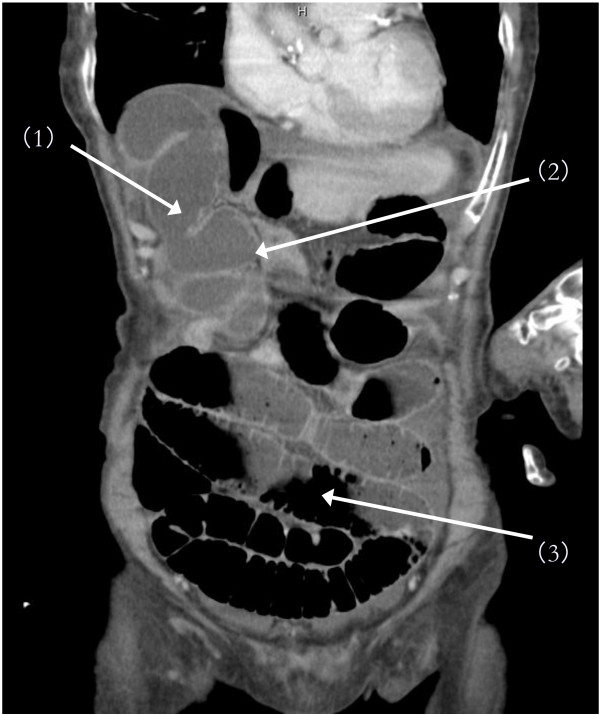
Pre-operative abdominal helical computed tomography (CT): coronal view. A membranous structure (1) was visualized in the center of the upper abdomen between the diaphragm and the left lobe of the liver, and dilated, edematous intestine (2) was seen on the right side of it, while air-dilated intestine (3) was seen on the left side and in lower abdomen.
